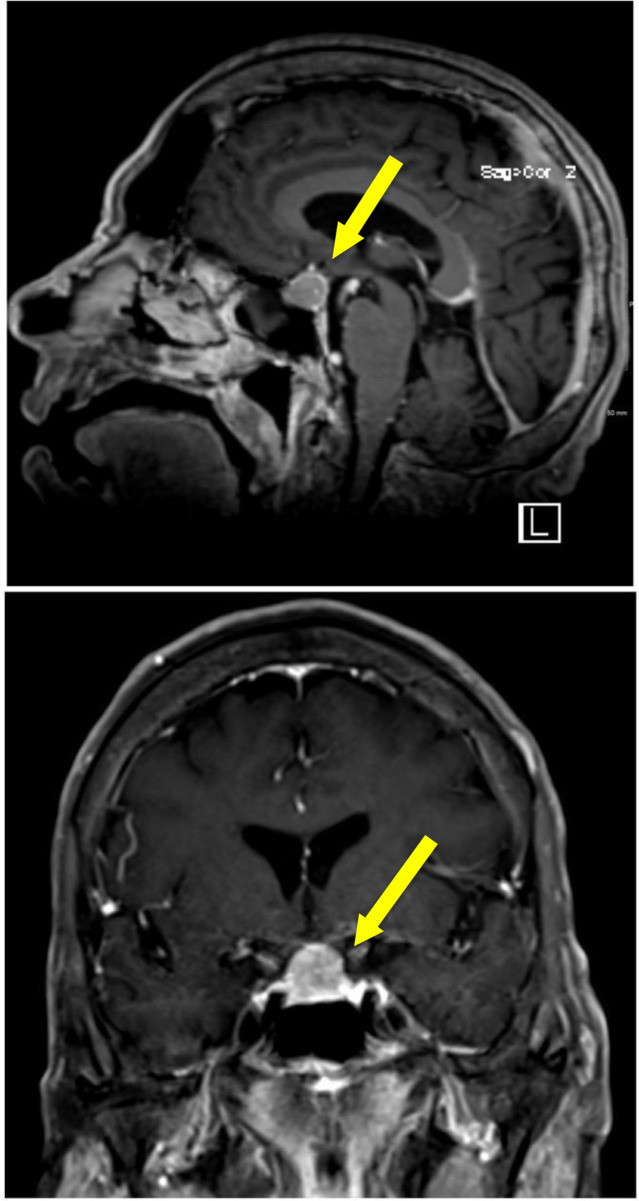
Sagittal and coronal MRI of the pituitary demonstrate homogeneously enhancing enlarged pituitary gland - pituitary macroadenoma.
